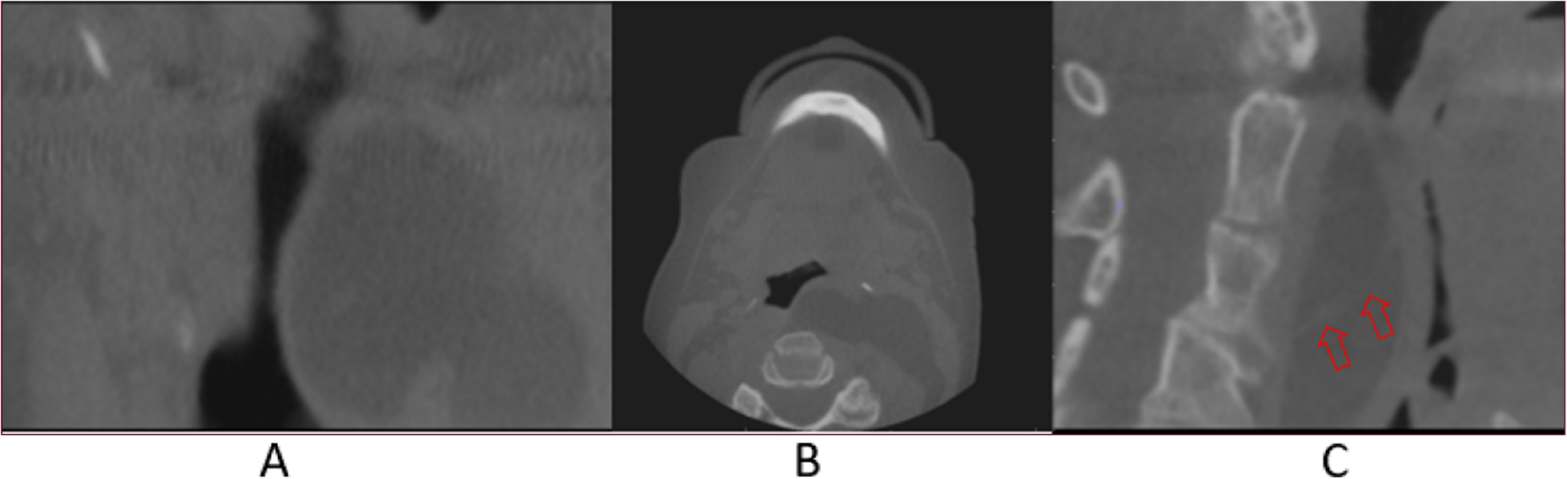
CBCT coronal (a), axial (b), and sagittal (c) images showing the soft tissue density lesion with faint internal septation (arrows). The full extent of the lesion was not initially within the field of view. No obvious change to the external soft tissue contours can be seen
